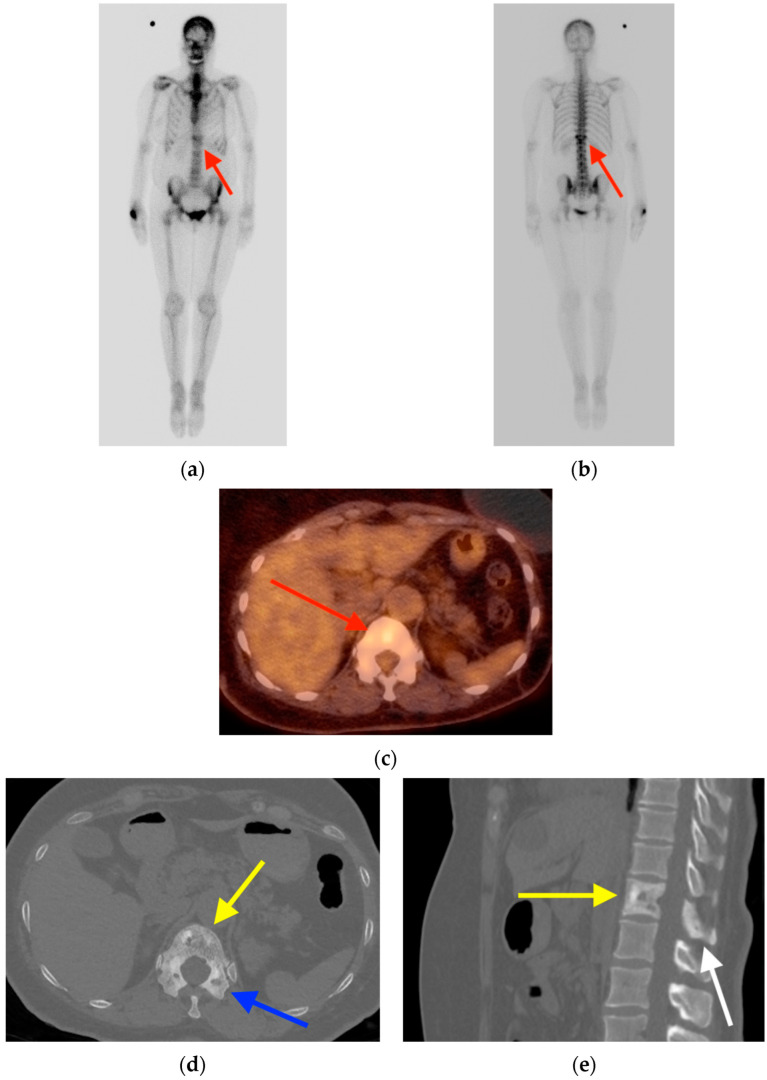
(a,b) Bone scintigraphy in a 64-year-old women with breast cancer shows focal uptake in the T12 vertebral body (red arrows). (c) FDG PET/CT also revealed focal radiotracer uptake (red arrow). Axial (d) and sagittal reformat (e) CT images show diffuse trabecular thickening involving the vertebral body (yellow arrows), pedicles (blue arrow), and posterior elements (white arrow), classic for Paget’s disease. CT = computed tomography. FDG PET/CT = fluorine-18 fluorodeoxyglucose positron emission tomography/computed tomography.
